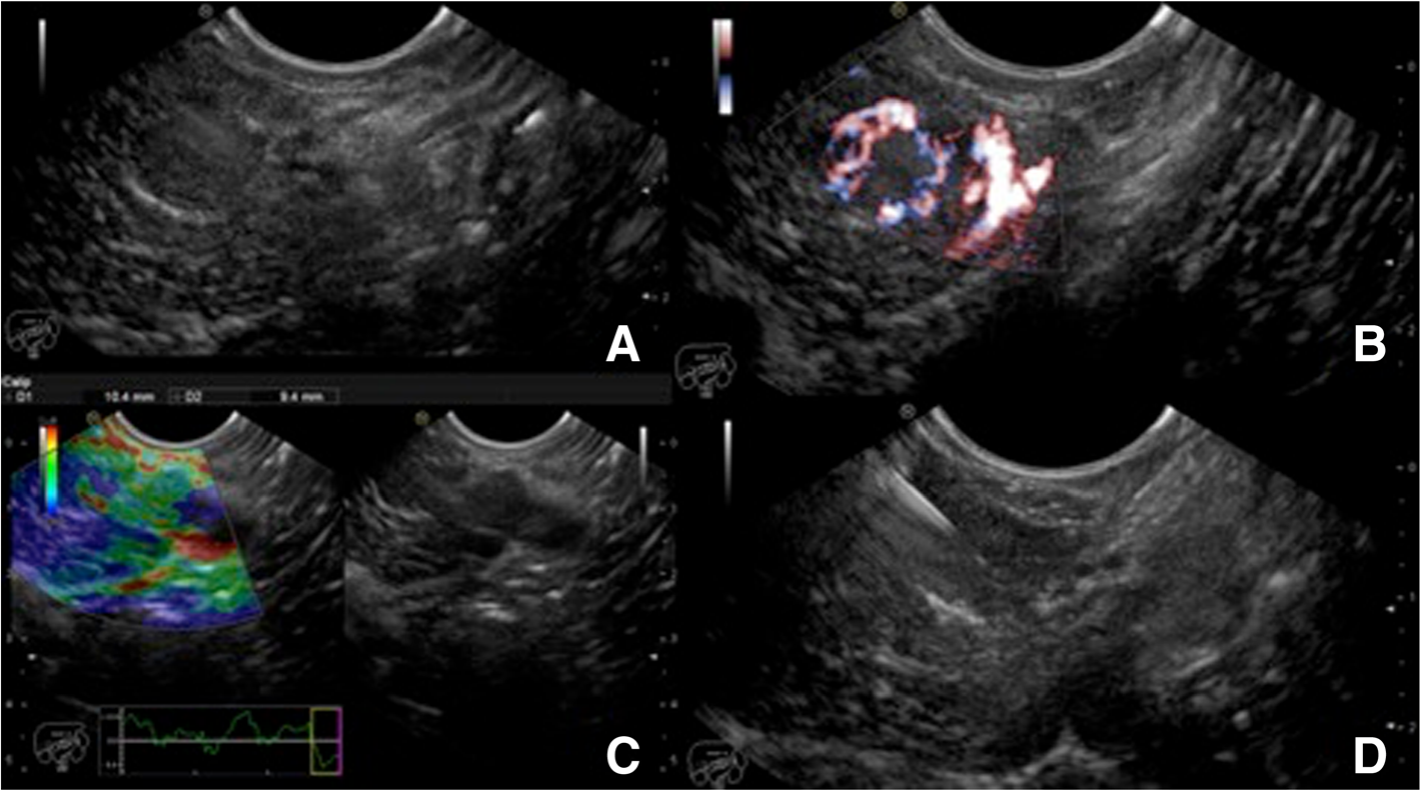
a: Endoscopic ultrasound (EUS) detection of solid hypoechogenic lesion with distinct boundaries in the tail of the pancreas. b: Color Doppler application revealing a hypervascular lesion. c: Elastography application revealing lower elasticity values compared to healthy pancreas. d: EUS-guided Fine Needle Aspiration (FNA) with a 25 G needle, yielding the final diagnosis of insulinomas
